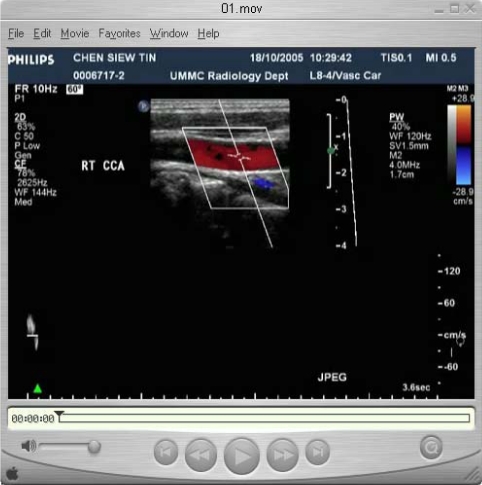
An example of a QuickTime movie showing a carotid Doppler ultrasound image (courtesy of Dr. A Vijayananthan).
